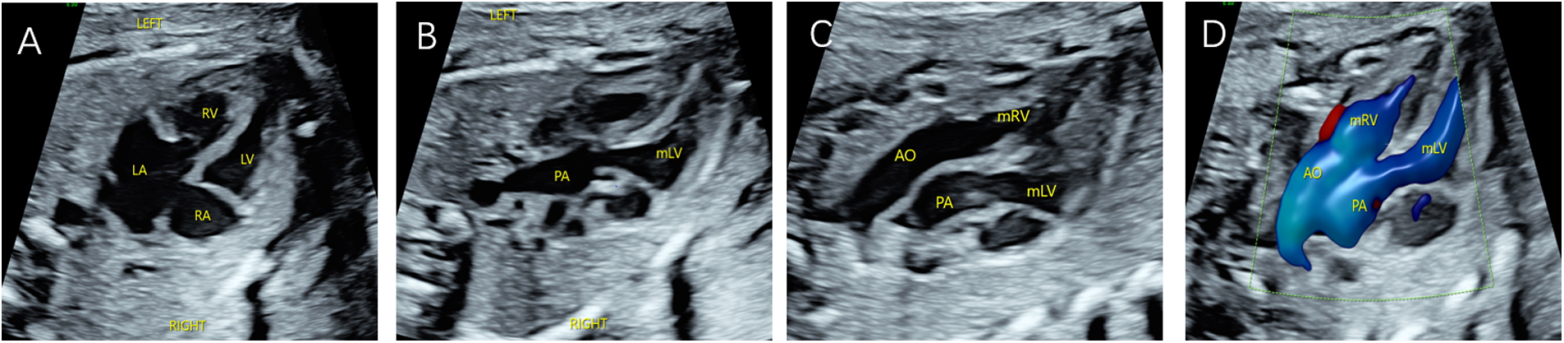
Fetal CC-TGA case confirmed by echocardiography at 23 weeks and 4 days. (A) Four-chamber heart view showing inconsistent atrioventricular connections, with the left atrium connected to the morphological right ventricle and the right atrium connected to the morphological left ventricle. (B–D) Right ventricular outflow tract view showing the pulmonary artery arising from the morphological left ventricle and left ventricular outflow tract view showing the aorta arising from the morphological right ventricle, with the aorta located anteriorly to the left of the pulmonary artery.
